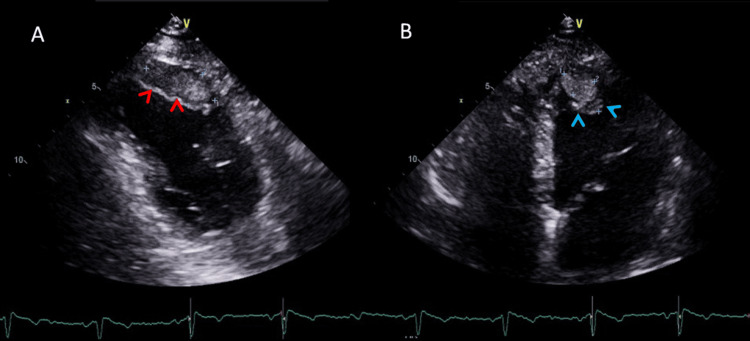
Ultrasound images of the cardiac chambers. (A) Parasternal view of the right ventricle showing an echogenic, slightly heterogeneous intramyocardial mass measuring approximately 17 × 36 mm (red arrowheads). (B) Four-chamber view revealing a second myocardial mass with similar radiologic characteristics, appearing as a slightly heterogeneous echogenic lesion within the apical aspect of the left ventricle, measuring approximately 15 × 23 mm (blue arrowheads).
